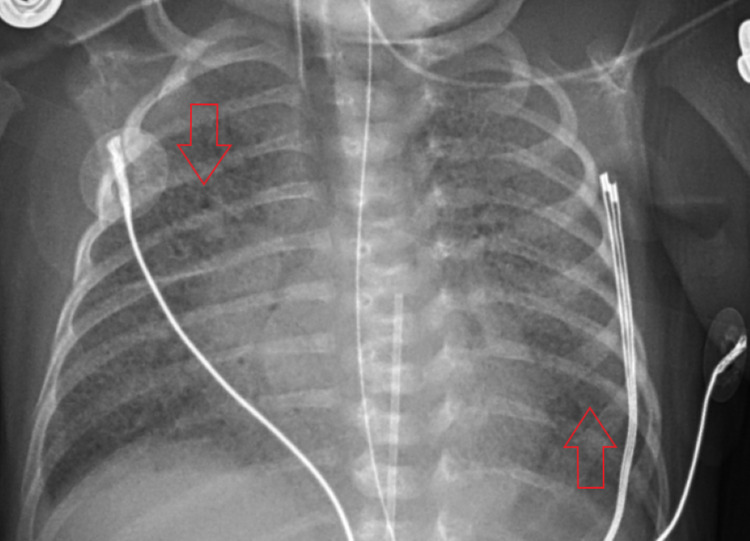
CXR from day 9 of life shows worsening of diffuse, bilateral granular infiltrates (arrows) CXR -  chest x-ray
